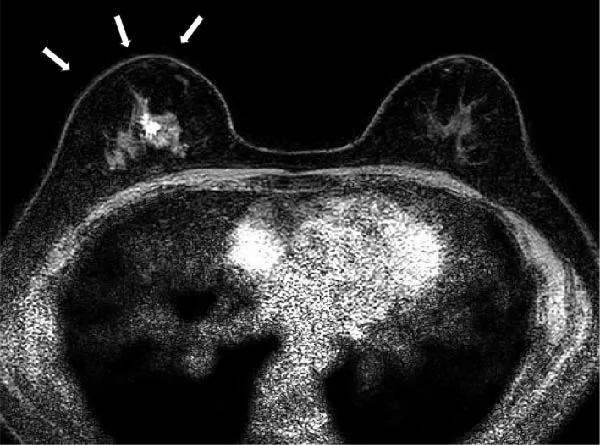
Magnetic resonance imaging. Contrast‐enhanced magnetic resonance imaging (MRI) of the breast showed an irregular mass in the upper‐outer and upper‐inner quadrants of the right breast, consistent with malignancy, and no axillary lymph node metastasis.
